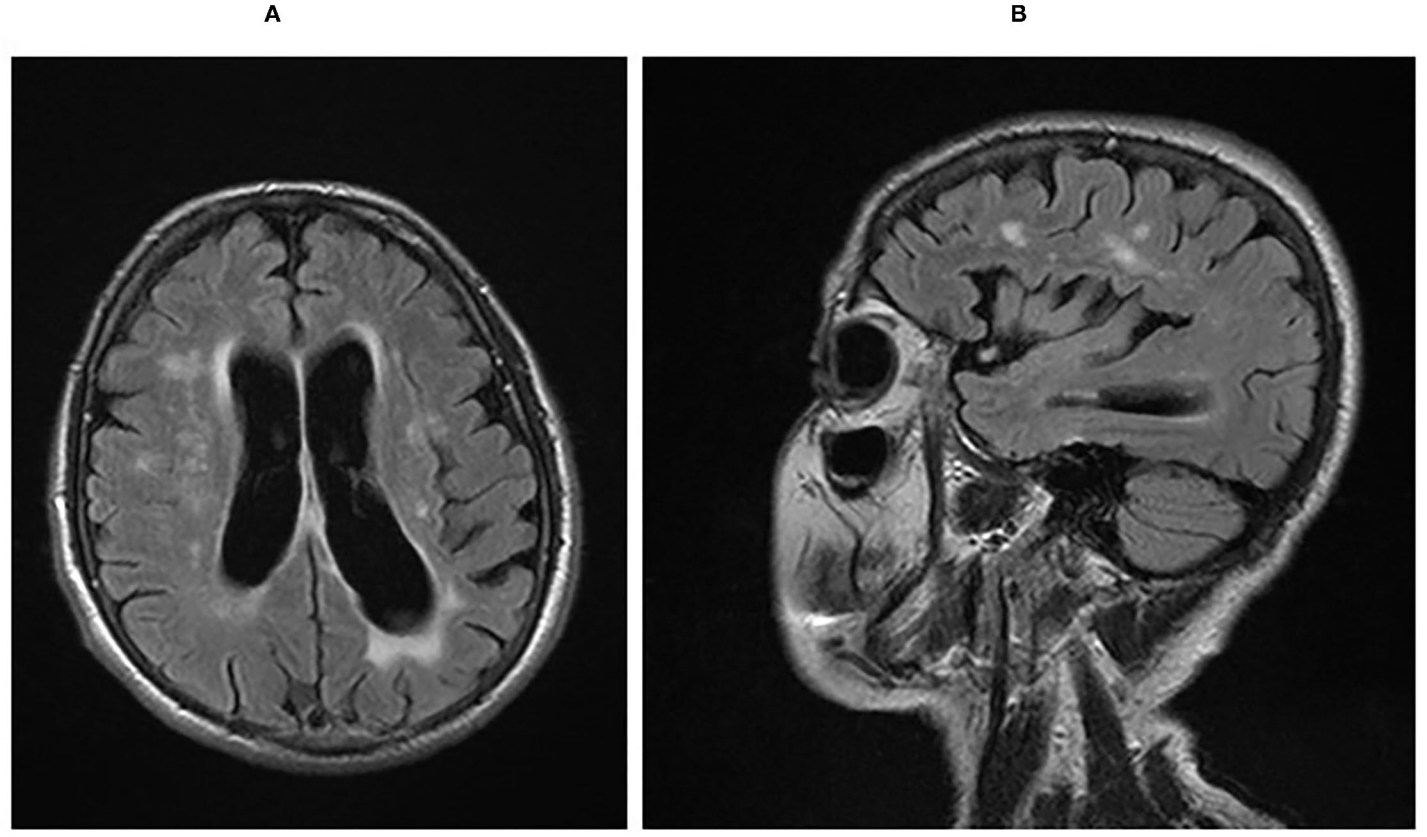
(A) Sagittal FLAIR MRI sequence showing cerebral atrophy with a frontotemporal predilection and post-ischemic hyperinsities in the white matter. (B) Axial FLAIR MRI sequence showing asymmetry of frontotemporal atrophy with left-side predominance. FLAIR, fluid-attenuated inversion recovery; MRI, magnetic resonance imaging.
